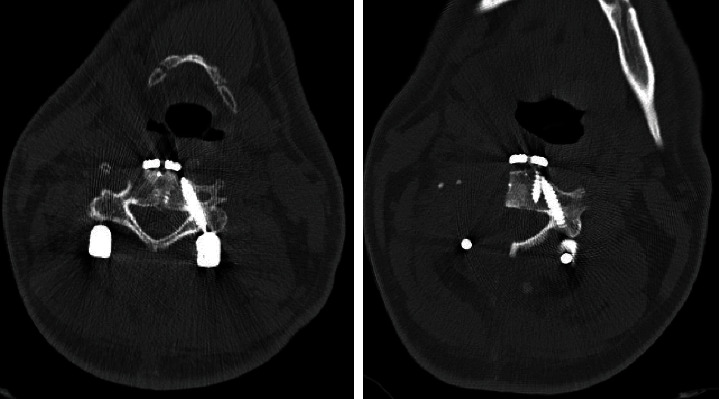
Precision of screw positioning. Axial reconstructions of the postoperative CT scan (case 2) to evaluate the correct placement of the pedicle screws. In both examples, the correct position of the screws could be confirmed.
